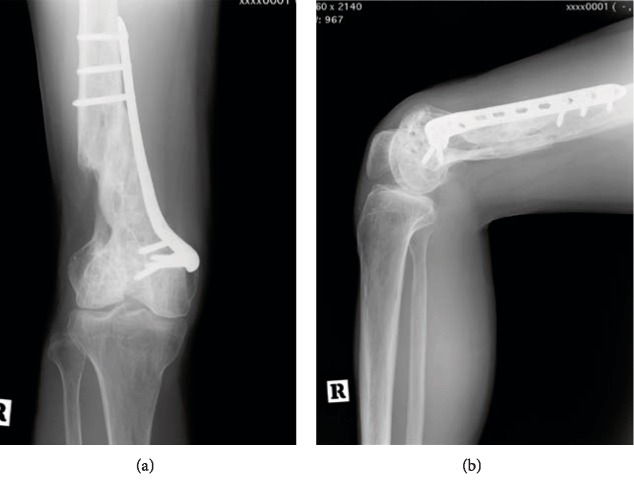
Radiograph of the knee at 2-year follow-up. Anteroposterior (a) and lateral (b) radiograph shows the union of the metaphyseal nonunion.
